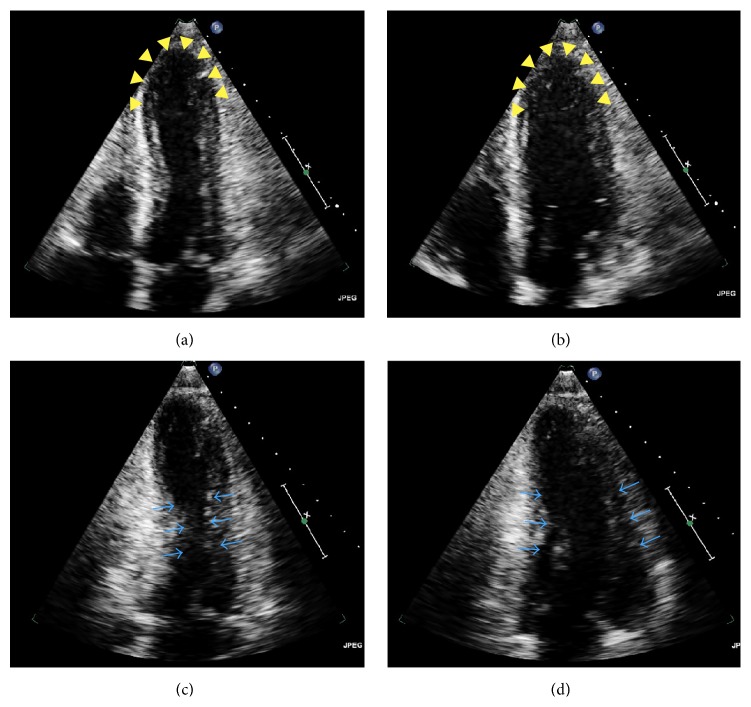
Initial transthoracic echocardiogram in the acute setting revealing features suggestive of stress cardiomyopathy. Systolic (a) and diastolic (b) apical 4-chamber views reveal akinetic apical and mid-anteroseptal segments (arrowheads) with hyperdynamic middle segments. Systolic (c) and diastolic (d) apical 2-chamber views disclose normal wall motion of the mid-cavity (arrows). The circumferential pattern of left ventricular myocardial dysfunction characterized by symmetric wall motion abnormalities involving the septal, anterior, and lateral walls is highly suggestive of stress cardiomyopathy.
